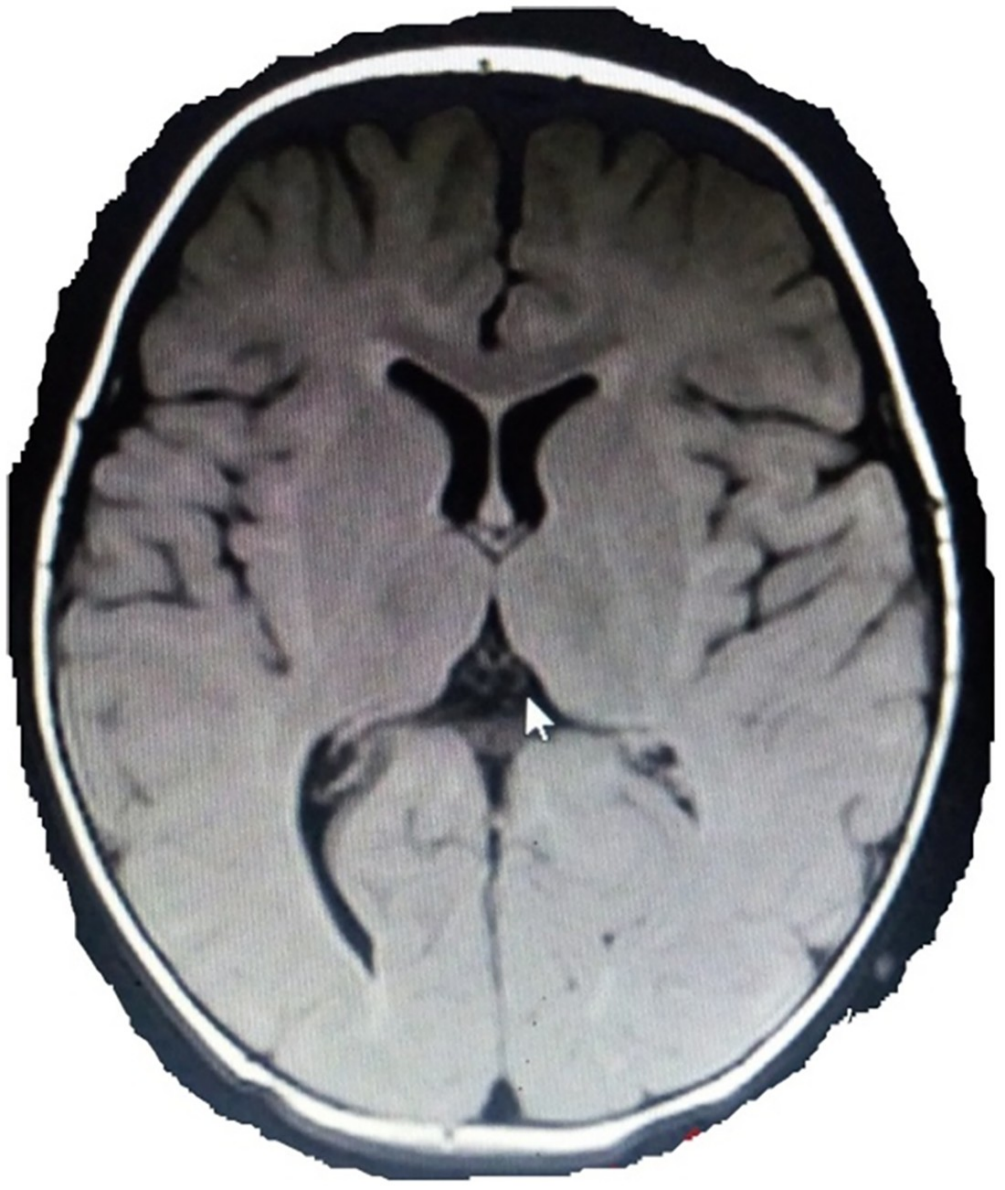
MRI of an 11 month old patient with spastic diplegia. MRI of an 11 month old male patient born at term with spastic diplegia (Case 31) showing deep right occipital horn posteriorly with temporal/frontal minimal increment in CSF spaces including cisterns (T2 FLAIR).
